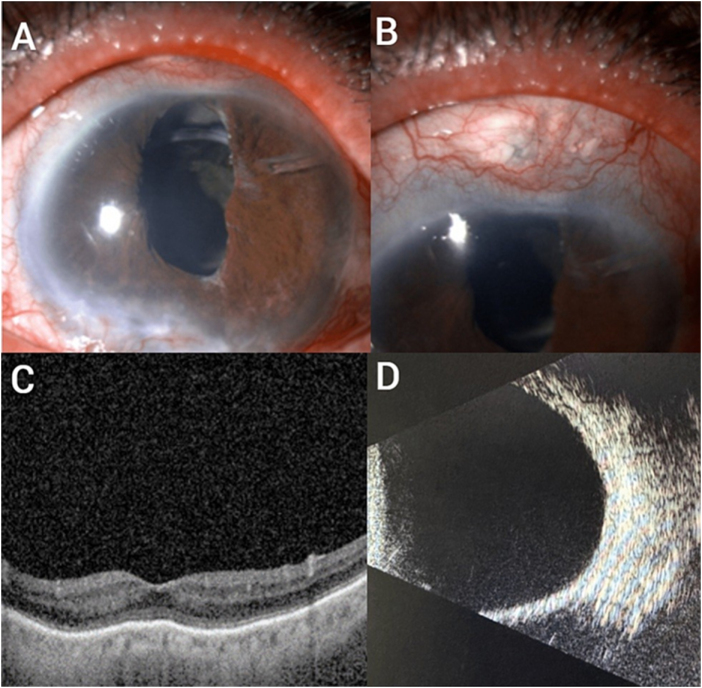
Slit-lamp photos of the anterior segment, optical coherence tomography macula, and B-scan ultrasound.
